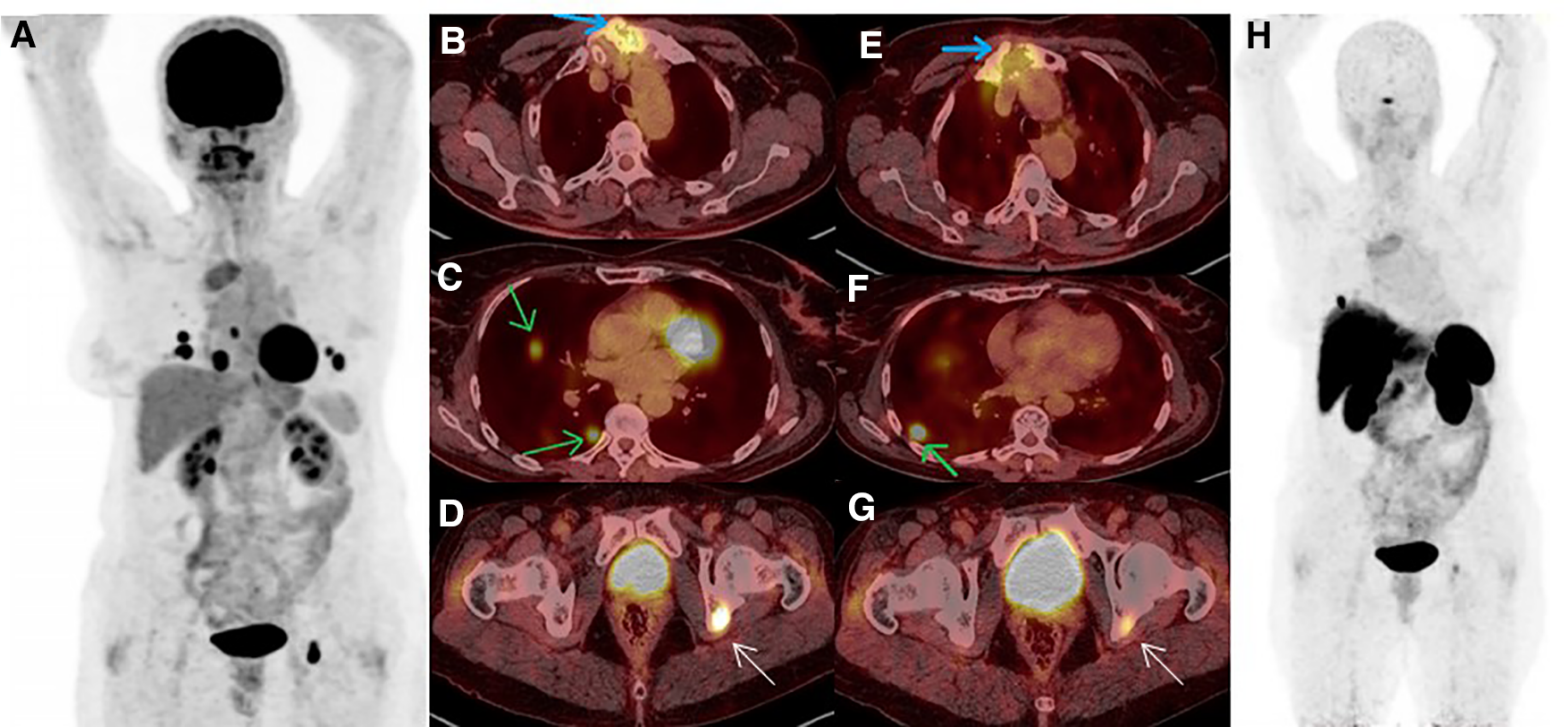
(A–D) ^18^F-FDG PET/CT MIP revealed increased tracer uptake in pulmonary nodules, sternal, and acetabular lesions (green, blue and white arrows, respectively). (E–H) A few days later, ^68^Ga-DOTA-TATE PET/CT confirmed somatostatin-receptor (SSTR) uptake in the above-mentioned areas, albeit most of the pulmonary nodules were non-SSTR-avid.
